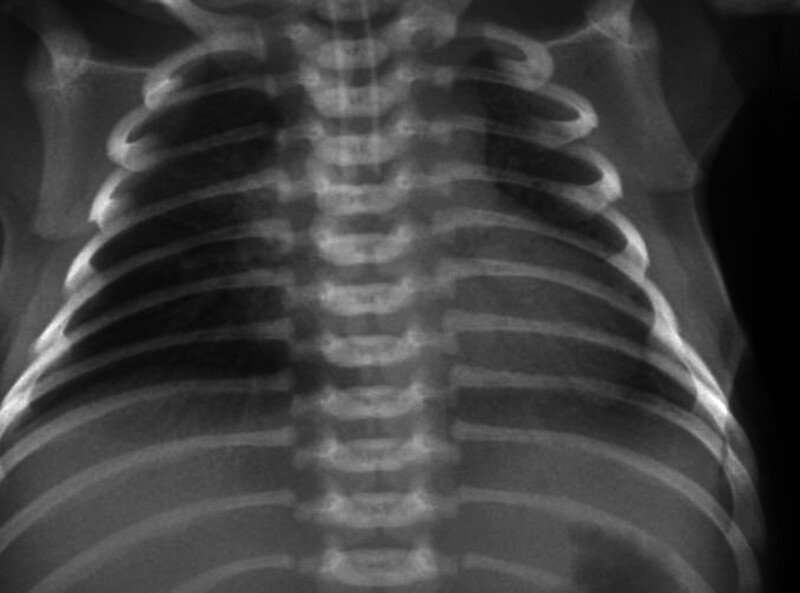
A chest X-ray review after intratracheal drip of lung surface-active substance suggests a slight increase in lung translucency on chest X-ray, proposing a slight improvement in lung perfusion.
